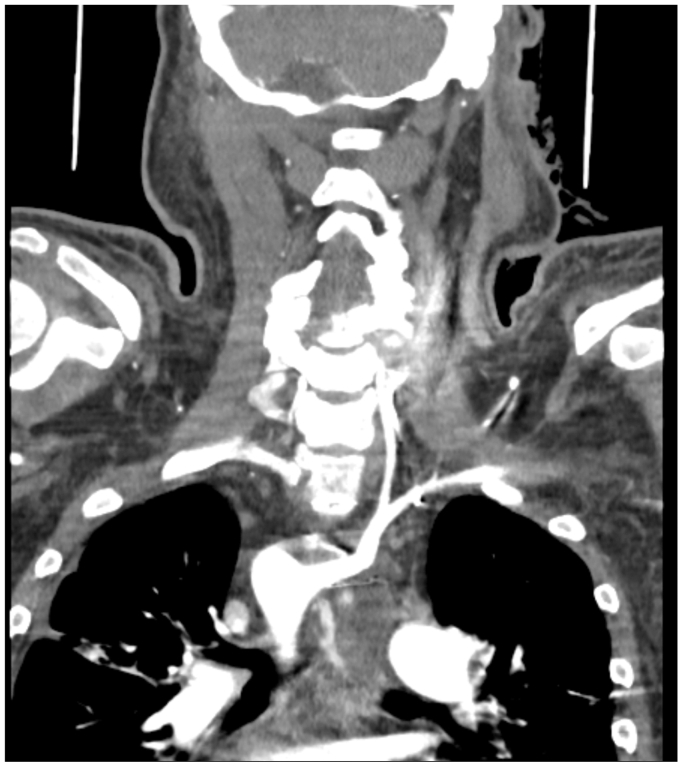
Coronal computed tomography angiography (CTA) showing the left subclavian artery-esophageal fistula.
